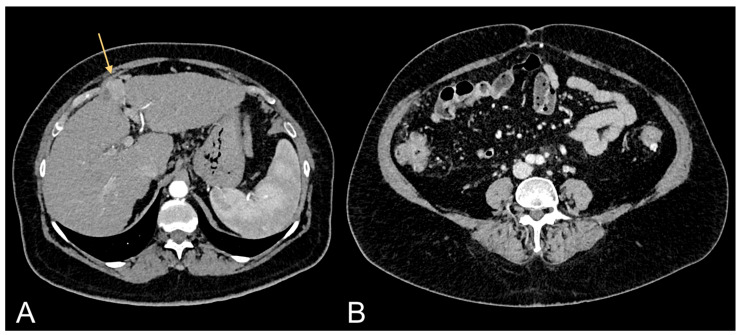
Contrast-enhanced CT images of a 69-year-old female patient showing HCC in cirrhotic liver (light orange arrow in (A)) and relative excessive amount of VAT (B).
